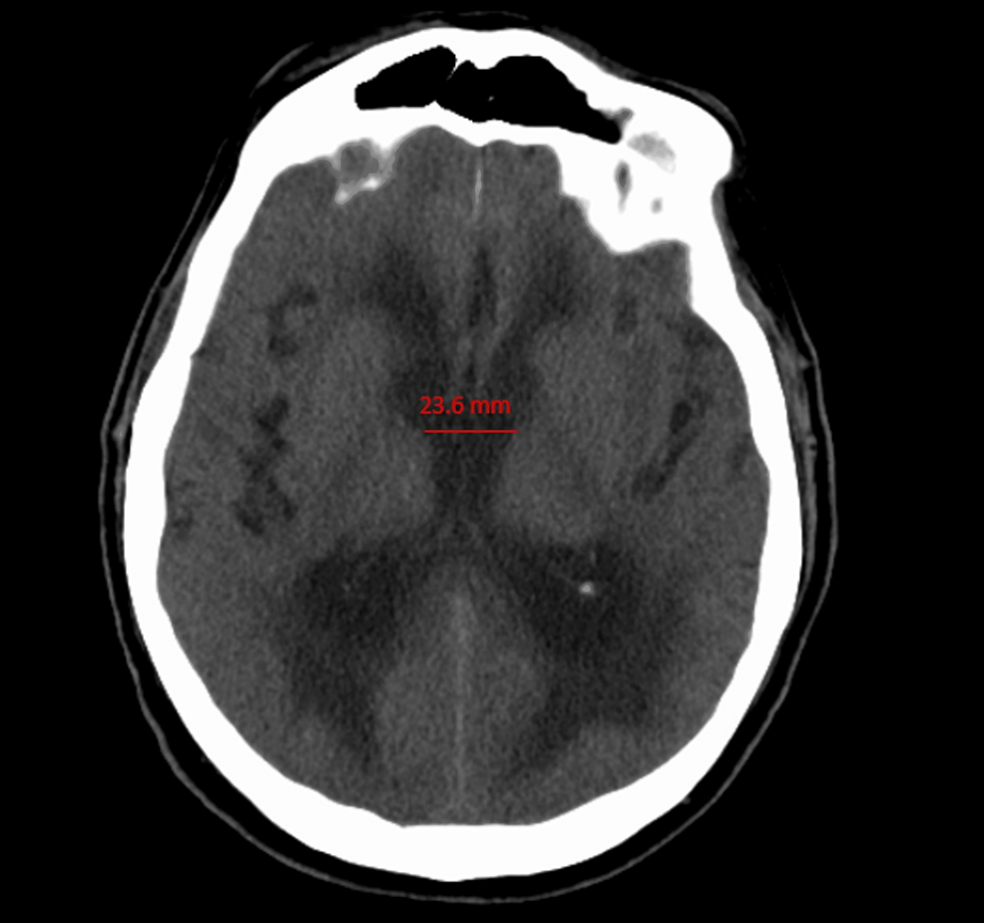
CT of the head without contrast after two weeks from the initial scan shows worsening of hydrocephalus, with increased width of the third ventricle from 19.2 mm to 23.6 mm.
